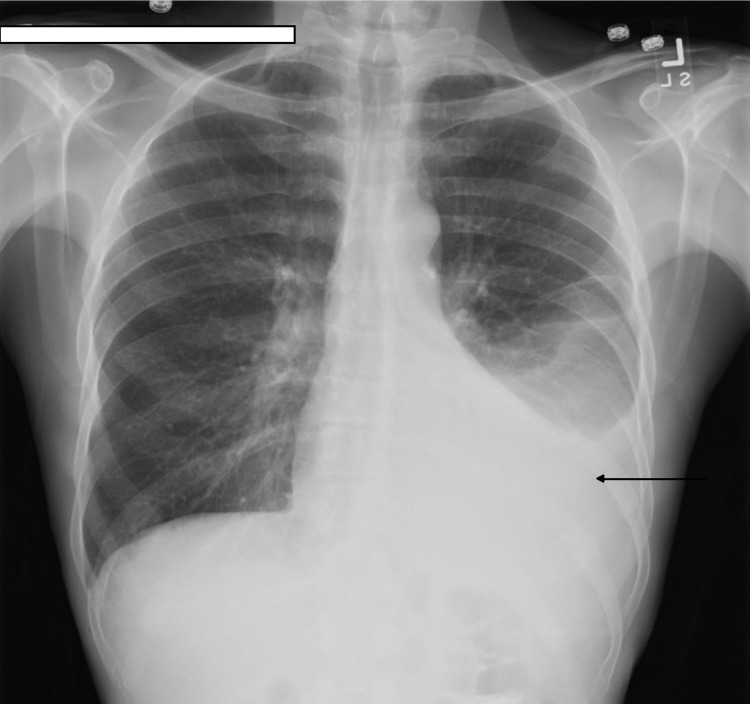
Chest X-ray shows large left side pleural effusion (arrow).
